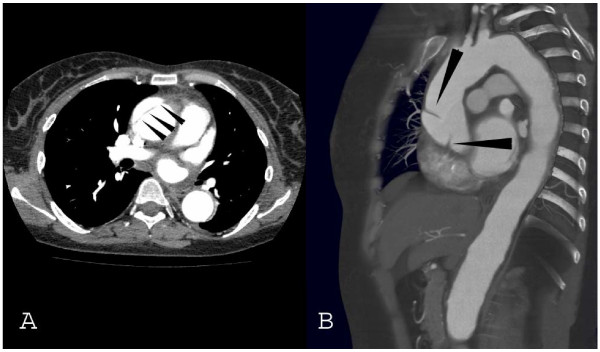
Transverse (A) and sagittal (B) CT views of the aorta. The dissection (arrows) is circumferential and begins distally to the coronary ostiae. arrows: dissection membrane
